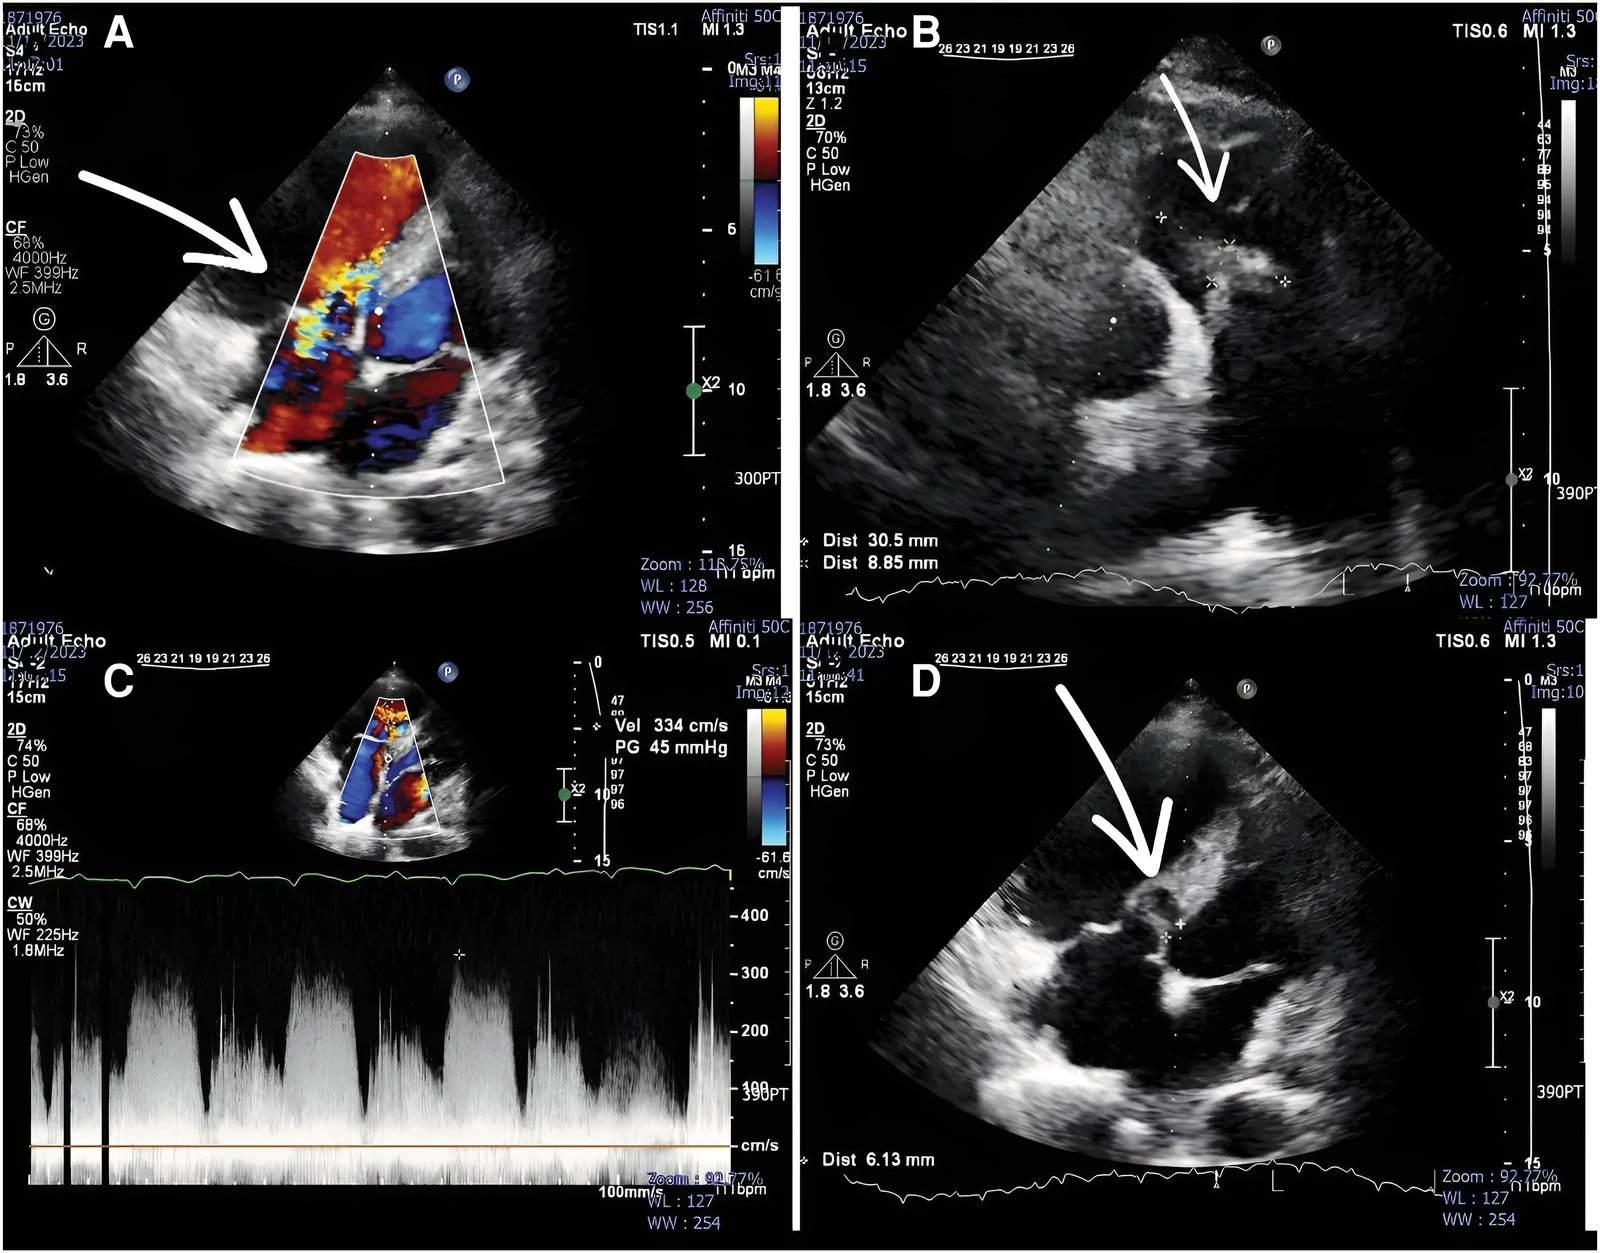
Transthoracic echocardiography (TTE) images demonstrating ventricular septal defect (VSD) and vegetations involving the pulmonary valve (PV). (*A*) Off-axis apical four-chamber view demonstrating a VSD with left-to-right shunt during systole. (*B*) Right ventricular outflow tract (RVOT) view showing the PV with multiple large, mobile vegetations attached to the valve leaflets. (*C*) Pulsed/colour Doppler interrogation of the VSD demonstrating bidirectional flow, with both systolic left-to-right shunt and diastolic flow across the defect. (*D*) Measurement of VSD size from the left ventricular (LV) side.
